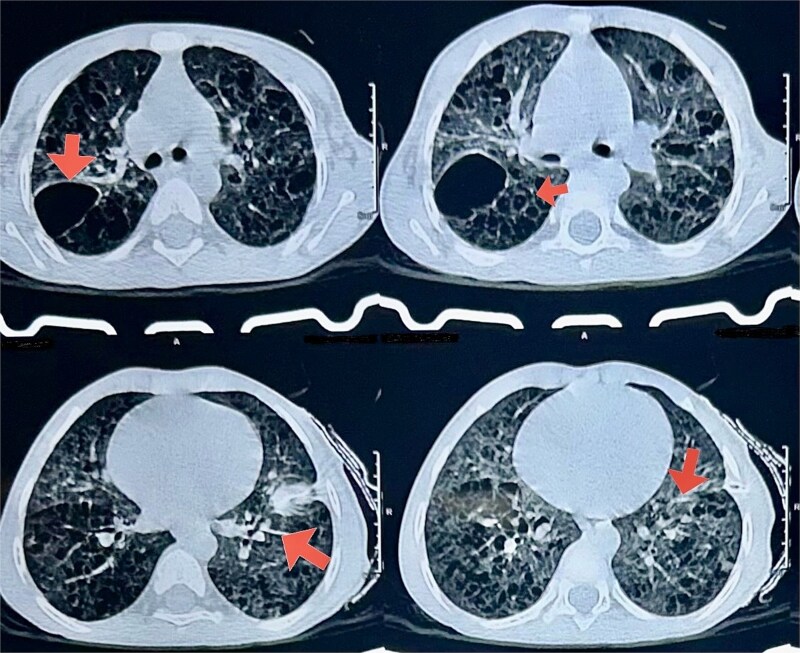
HRCT showing diffuse cystic lung disease.
